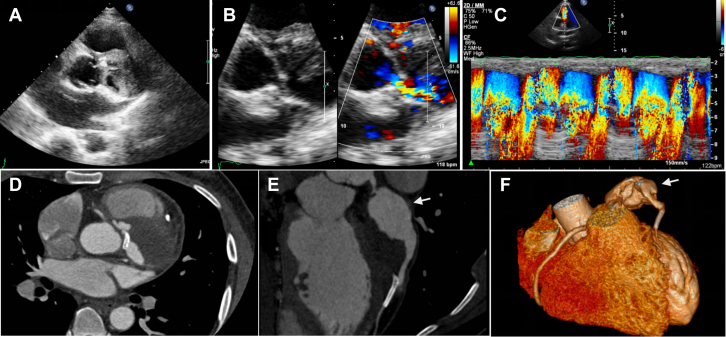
Case 2: Left Anterior Descending Infected Pseudoaneurysm With Right Ventricular Outflow Tract Communication (A and B) Parasternal short-axis echocardiographic views showing a pseudoaneurysmal cavity adjacent to the left coronary sinus and left main region. (C) Color M-mode image demonstrating continuous flow from the pseudoaneurysm into the right ventricular outflow tract. (D and E) Contrast-enhanced computed tomography images showing pseudoaneurysm arising from the stented left anterior descending artery segment. (F) Three-dimensional reconstructed computed tomography image showing the pseudoaneurysm in relation to the right ventricular outflow tract (white arrow).
